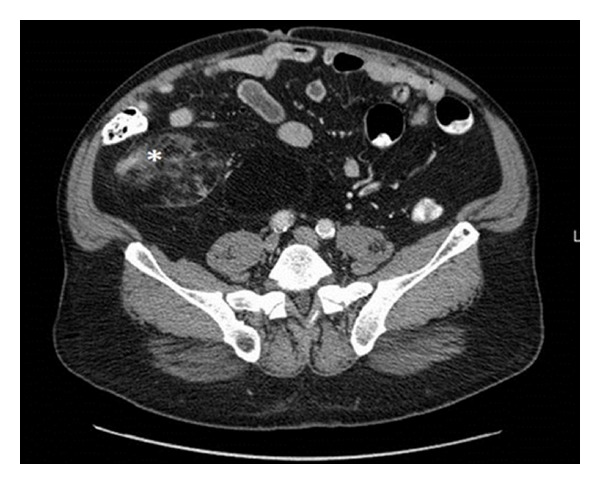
CT abdomen and pelvis with IV contrast, transverse image, displaying a large fatty lesion with associated soft tissue component (starred) in the right peritoneum anterior to the iliopsoas muscle. There is displacement of the bowel loops anteriorly and to the left.
